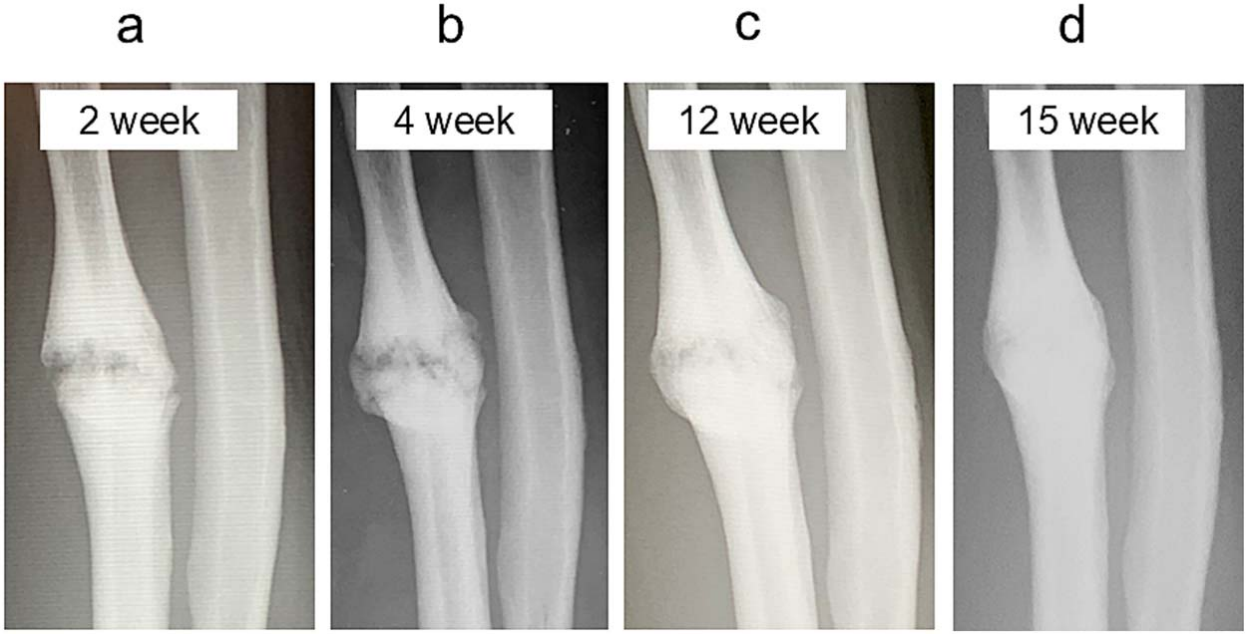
Radiographic findings during follow-up. (a) At 2 weeks, early callus formation is observed. (b) At 4 weeks, increased callus formation is noted. (c) At 12 weeks, near complete union is evident. (d) At 15 weeks, complete union achieved.
